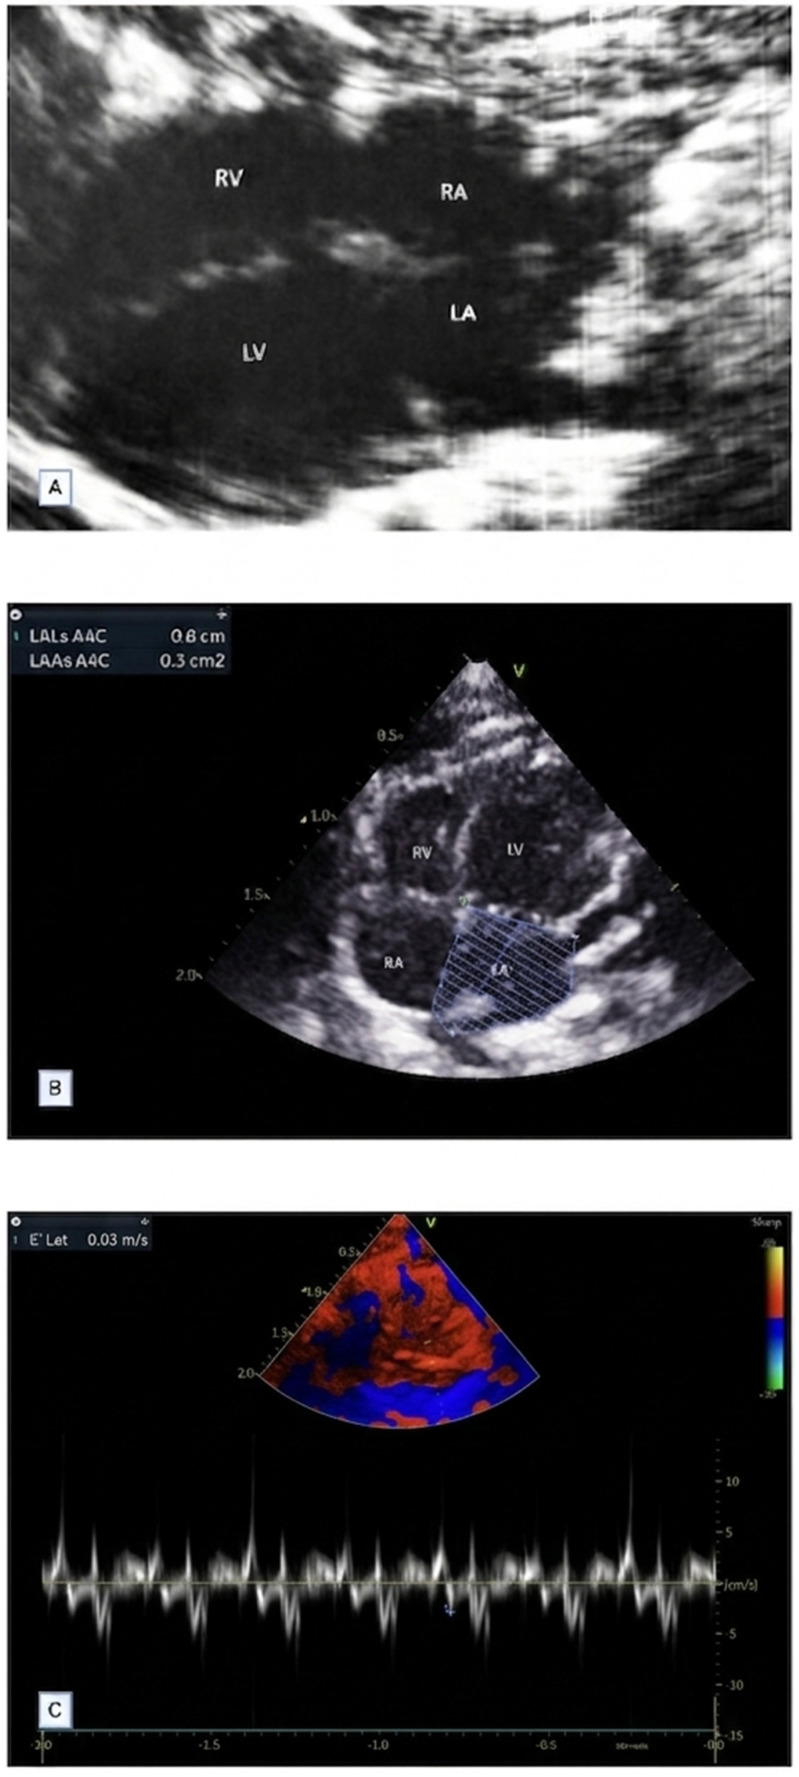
Echocardiography assessment. (A) Basic 4 chamber view. (B) LA area measurement. (C) E lateral.
